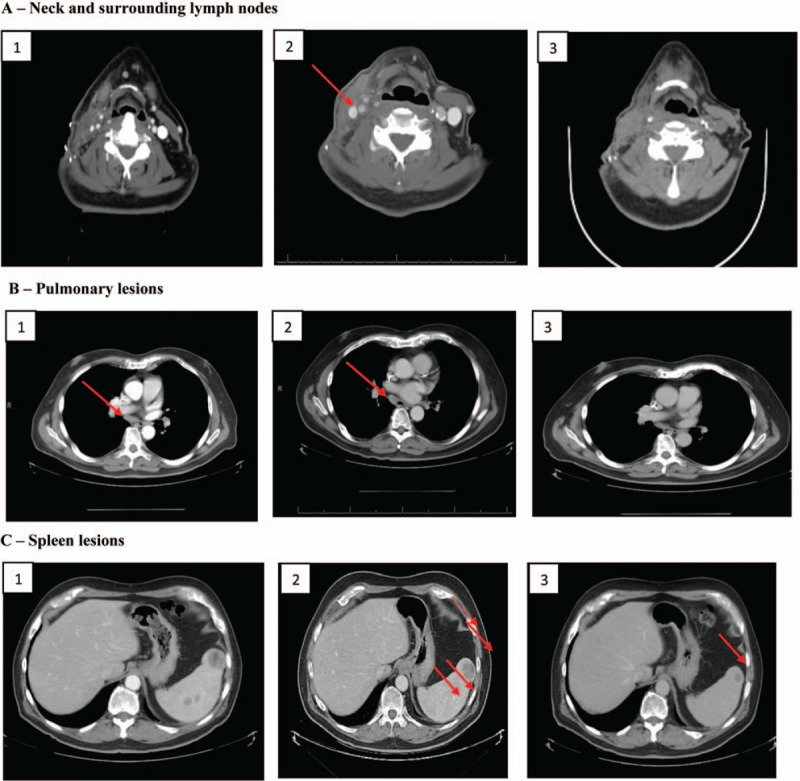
Response to therapy monitored by computed tomography. (A) Neck lesion and surrounding lymph nodes: (1) Before BRAFi treatment (January 2014). (2) Recurrence of melanoma after BRAFi treatment (December 2014). (3) After anti-CTLA-4 treatment (April 2015). (B) Pulmonary lesions: (1) After nivolumab and before pembrolizumab treatment (August 2015). (2) During pembrolizumab treatment (March 2016). (3) During pembrolizumab treatment (April 2018). (C) Spleen lesions: (1) Before pembrolizumab treatment (October 2015, size 3.5 cm). (2) During pembrolizumab treatment. (March 2016, size 3 cm). (3) During pembrolizumab treatment (April 2018, size 1.5 cm).
